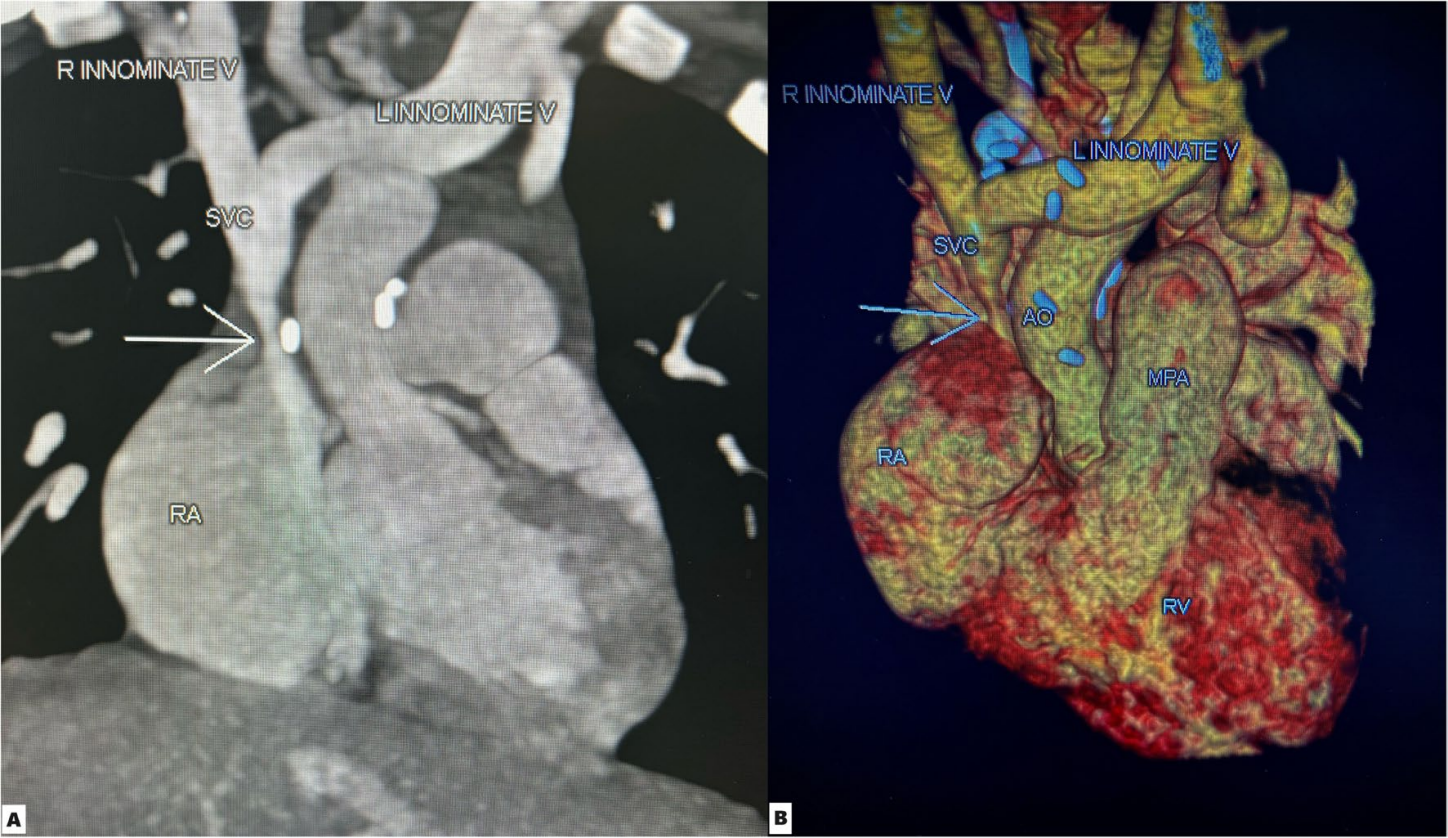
CT angiogram images of coronal view (A) and 3D reconstruction (B) showing narrowing at lower end of SVC
